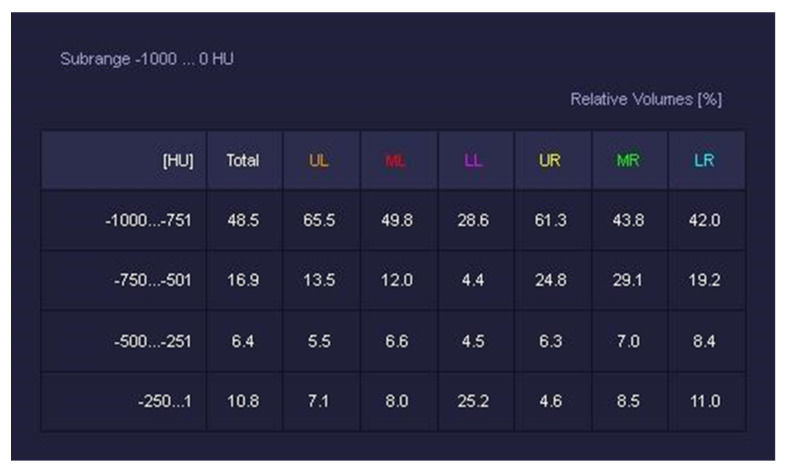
CT Pulmo 3D: thresholds and volumes. This figure shows the HU thresholds and relative volumes per lobe.
